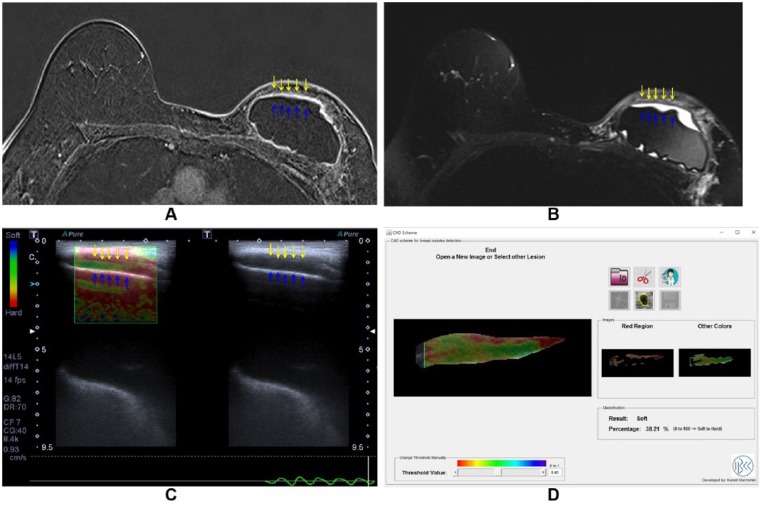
A 42-year-old woman breast augmentation surgery 3 years ago. (A) Axial T2-weighted sequence with silicone suppression presents collection between the fibrous capsule (yellow arrows) and the elastomer of the implant (blue arrows). (B) In the dynamic sequence, there is no enhancement of this collection corresponding to fluid collection. (C) By elastography, it is observed to correspond to soft lesion. (D) Elastography report.
